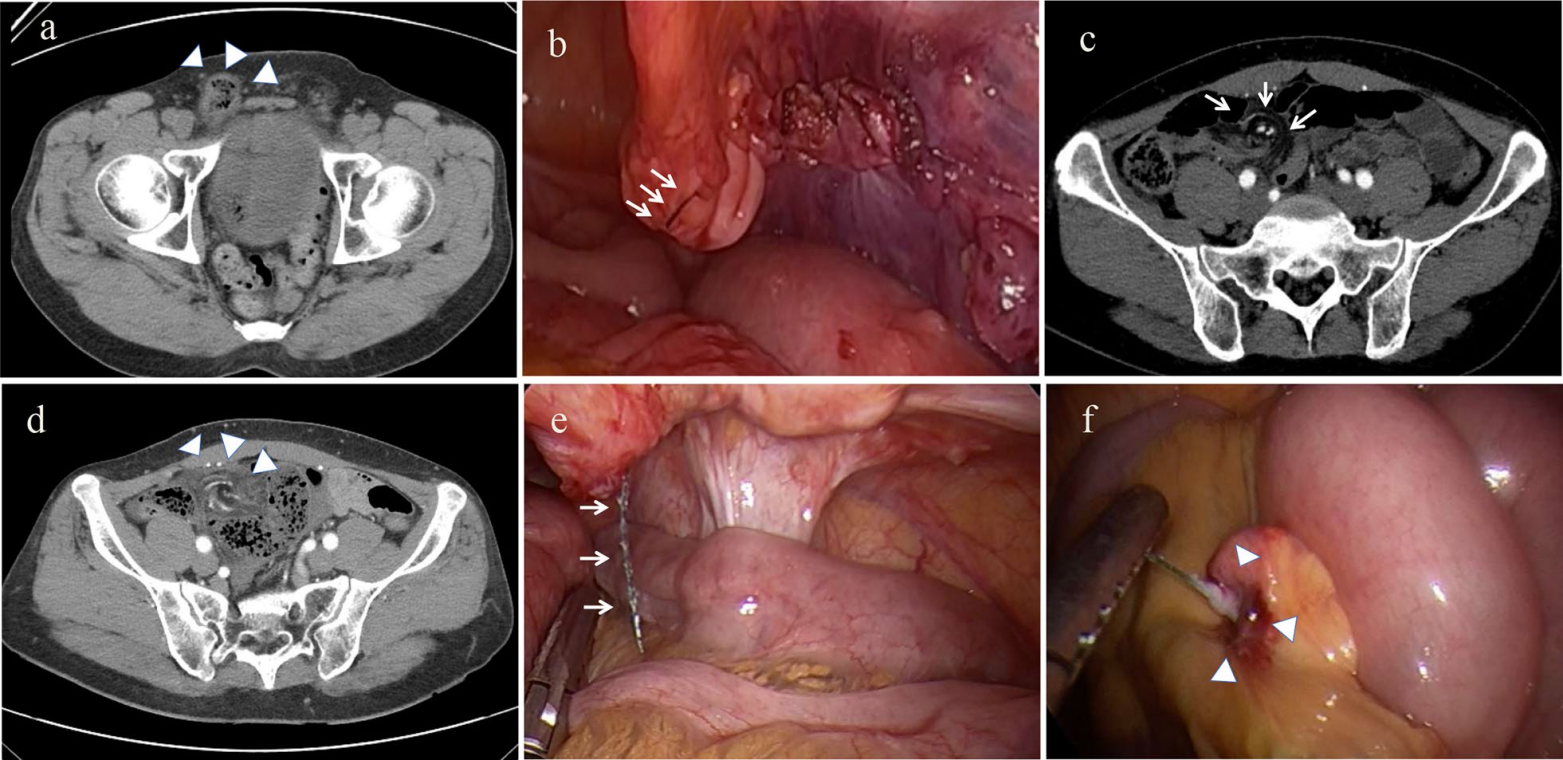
Preoperative and intraoperative images in Case 1. a CT shows right indirect inguinal hernia in prone position (white arrowheads). b A self-anchoring barbed suture device is used to close the peritoneal defect from the right side to the left. The residual tail is about 5 mm (white arrows). c Upon readmission due to vomiting on postoperative day 3, the CT scan shows a small bowel obstruction with possible volvulus of ileum (white arrows). d A small bowel obstruction with strangulation one month later (white arrowheads). e The tail of the barbed suture is much longer and the bowel segment is strangulated (white arrows). f The tail of barbed suture attached to the mesentery of the distal ileum (white arrowheads)
